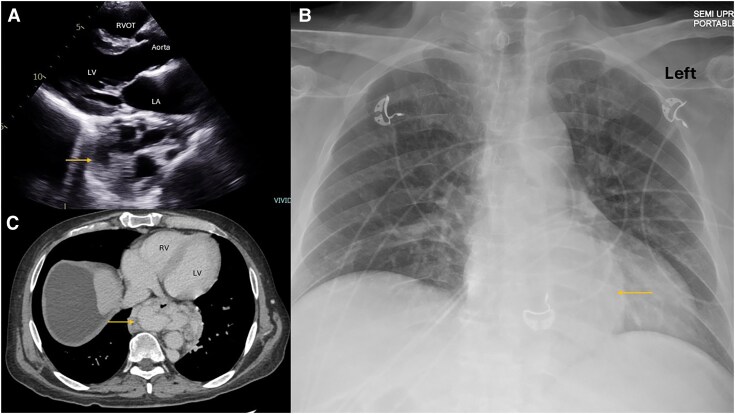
Parasternal long-axis view (*A*) showing a large well-circumscribed mass with cavitations posterior to the left atrium and basal left ventricle. Antero-posterior chest X-ray (*B*) revealed a large, rounded mass (arrow) located behind the cardiac silhouette. Computed tomography chest transverse view (*C*) showing a large multinodular-appearing mass (arrow) behind the left atrium and basal left ventricle. These are large oesophageal varices in a patient with hepatic cirrhosis and porto-pulmonary hypertension. LV, left ventricle; LA, left atrium; RVOT, right ventricle outflow tract.
